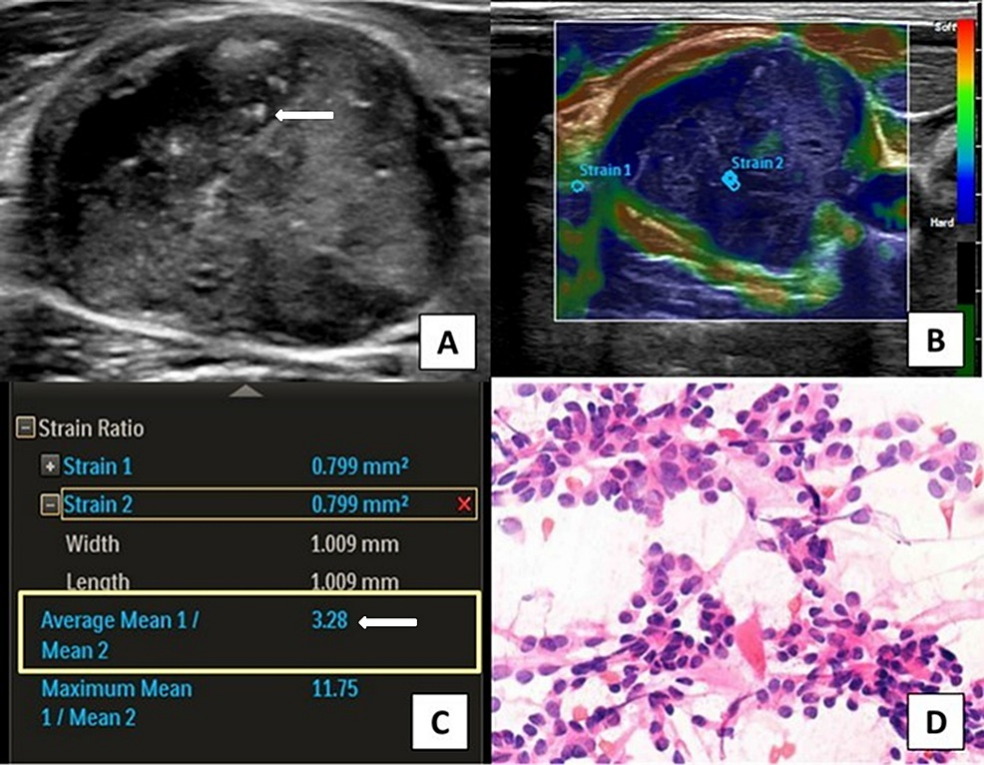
Ultrasound neck of 40-year-old male showing TIRADS 5 lesion in right lobe of thyroid and ipsilateral cervical lymphadenopathy (A) On USG, lymph nodes have multiple intranodal calcifications (arrow) with heterogenous echotexture. (B and C) On elastography, the lymph node shows pattern 5 with SI of 3.28 (arrow). Lymph nodes are diagnosed as malignant based on USG and elastography findings. (D) FNAC of right thyroid lesion and ipsilateral CLN (level 4) show papillary thyroid carcinoma with ipsilateral cervical nodal metastasis. TIRADS: thyroid imaging reporting and data system; USG: ultrasonography; SI: strain index; CLN: cervical lymph nodes; FNAC: fine-needle aspiration cytology
